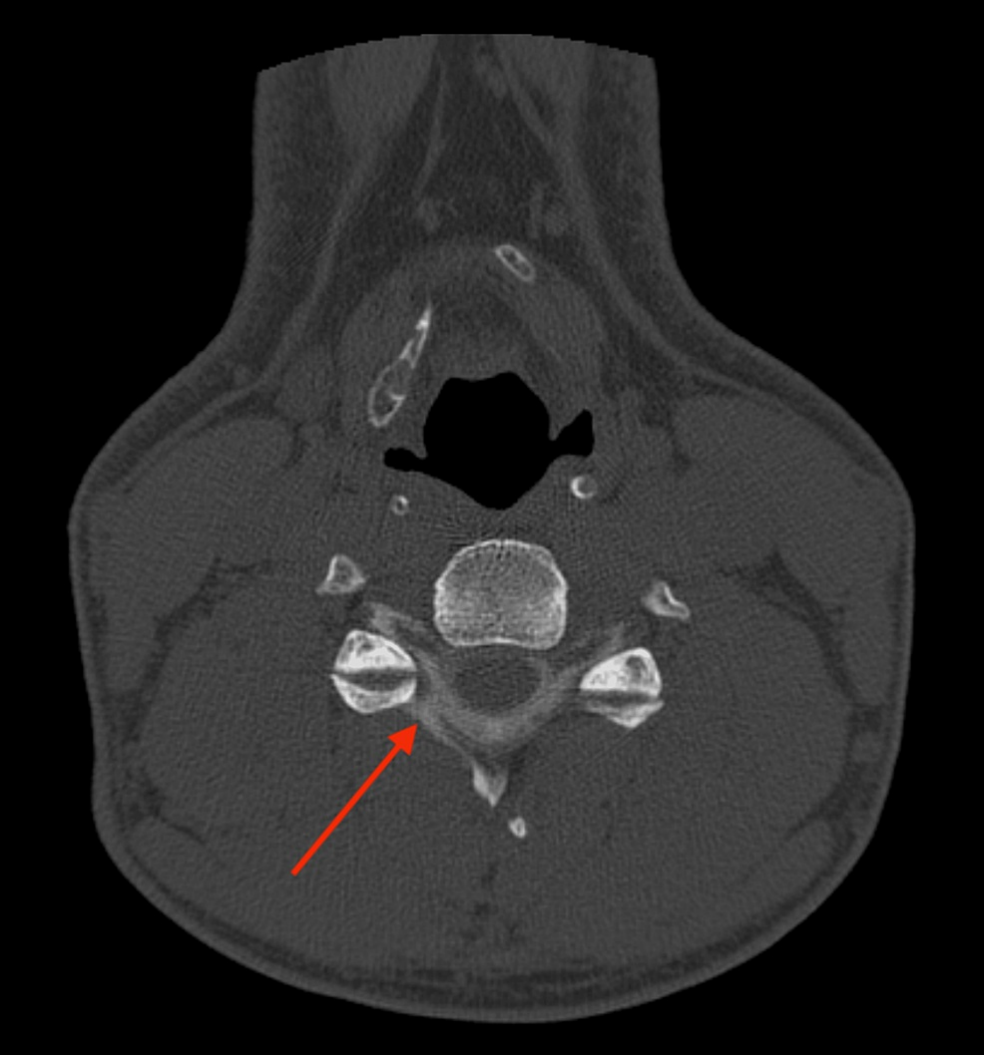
Axial C4-C5 level myelo-CT showing contrast outside the subarachnoid space (red arrow), as evidence of a right-sided C4-C5 CSF fistula.
